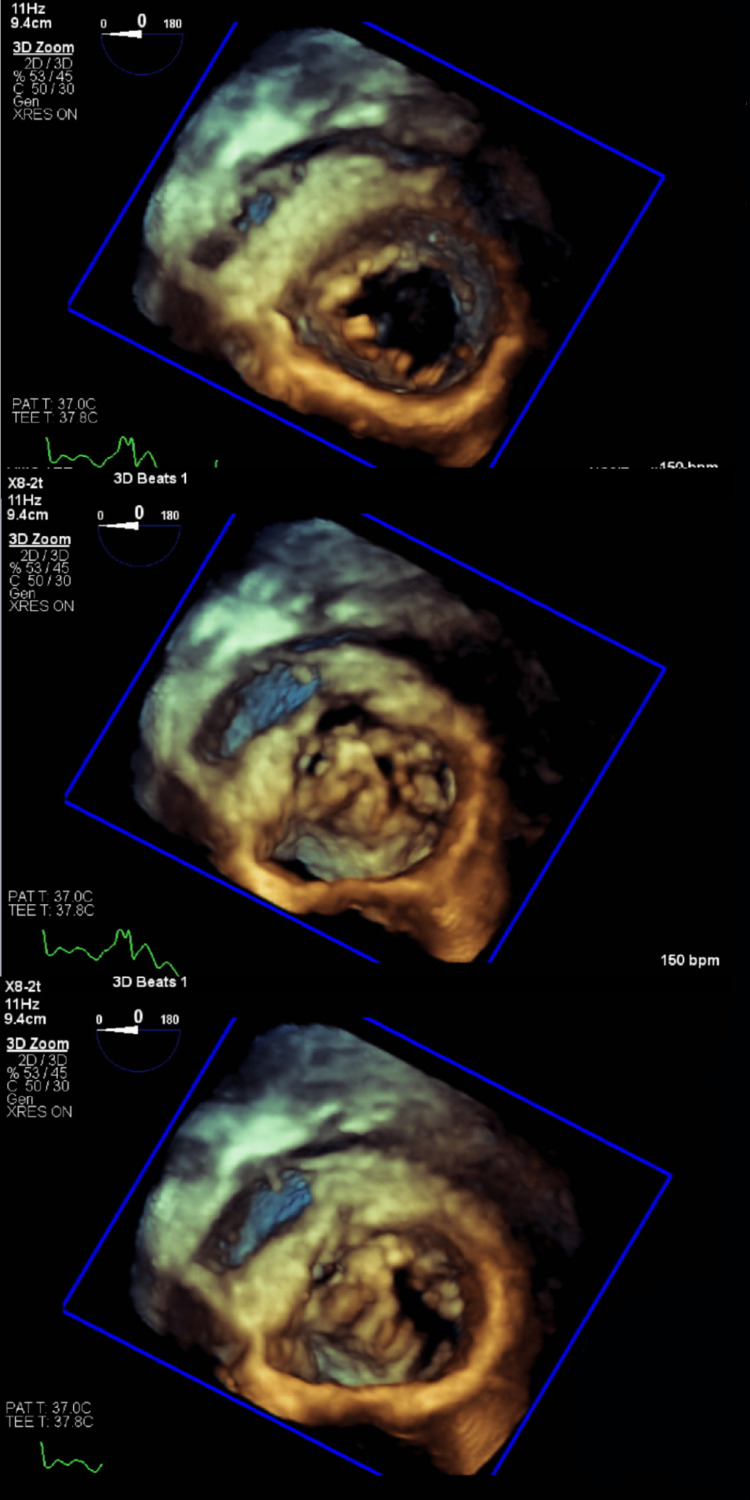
Transesophageal echocardiogram (TEE) showing mitral valve vegetations.
